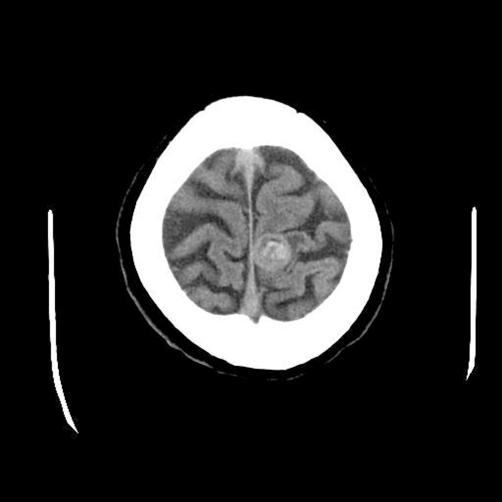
Brain CT. A solitary tumorous lesion (2 cm) is seen in the right temporal lobe. The tumor was solitary and well defined. No infiltrative growth was recognized. The density was that of blood. No edema was seen in the surrounding brain. The ridiologists’ diagnosis was cavernous hemanigioma.
